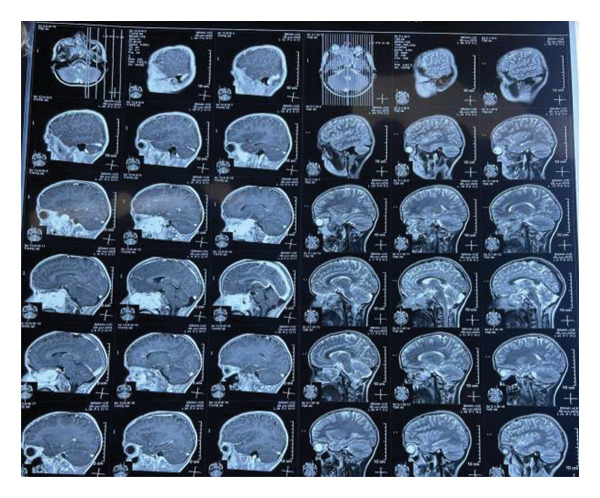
MRI brain with contrast sagittal view reveals the intracranial extension of the mass into the right middle cranial fossa, where it abuts the dura mater along the medial aspect of the right temporal lobe, resulting in a mass effect on the right temporal lobe. Additionally, it closely abuts the right cavernous sinus, with areas of extension into the sinus. However, the flow void of the right internal carotid artery (ICA) remains intact, suggesting no significant vascular occlusion or invasion.
